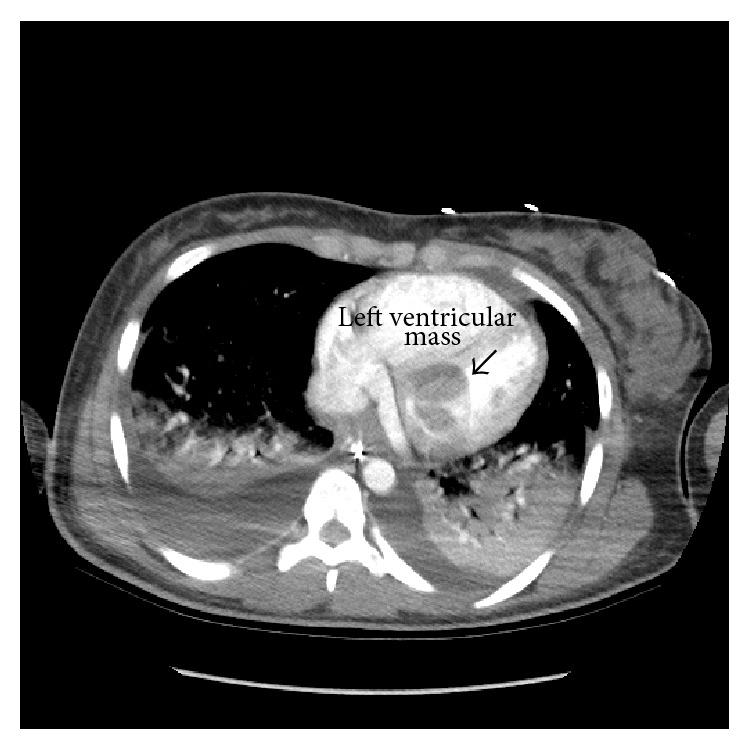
CT of the chest following intravenous contrast showing part of the mass extending into the left ventricular.
